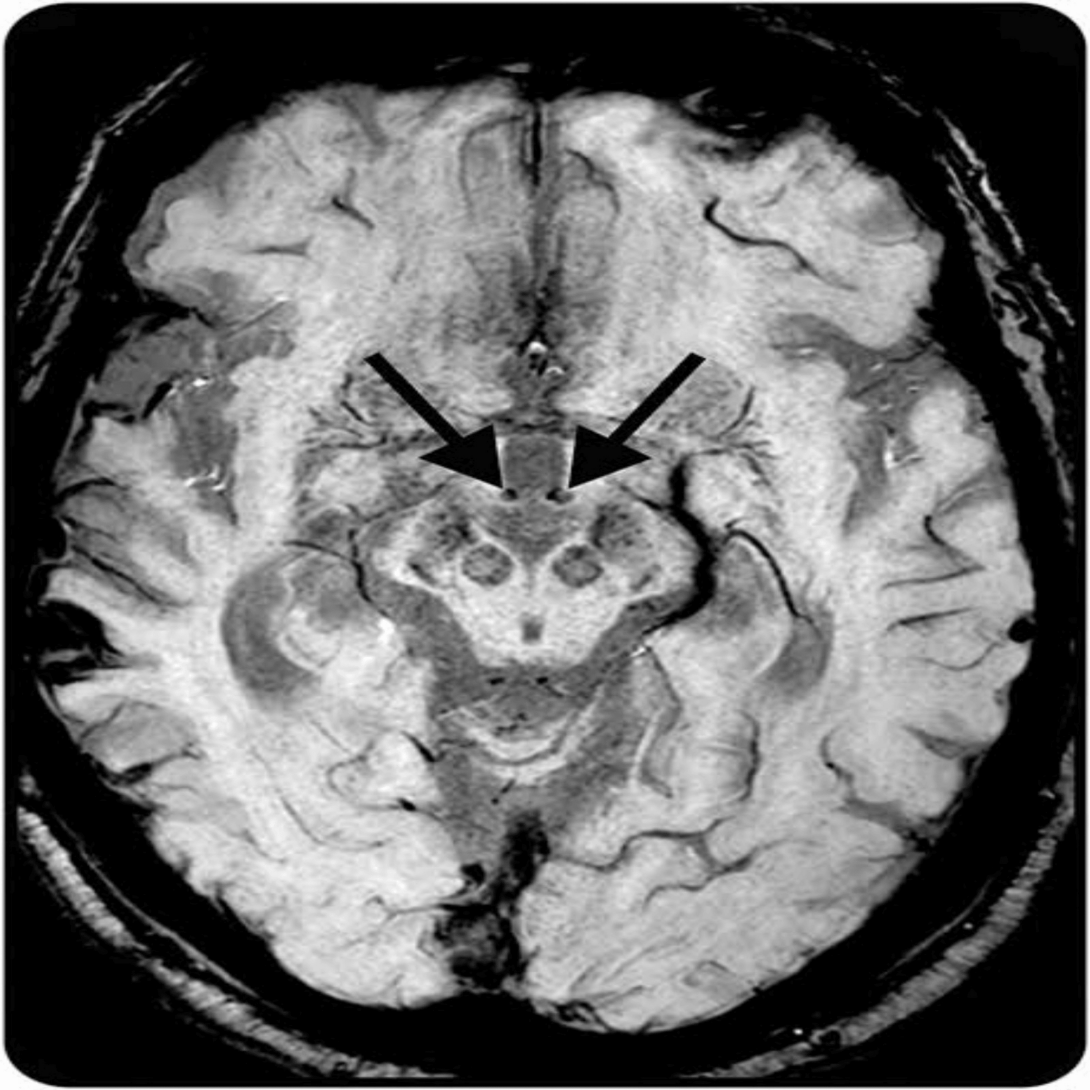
T2-weighted MRI image showing features of Wernicke’s encephalopathy
